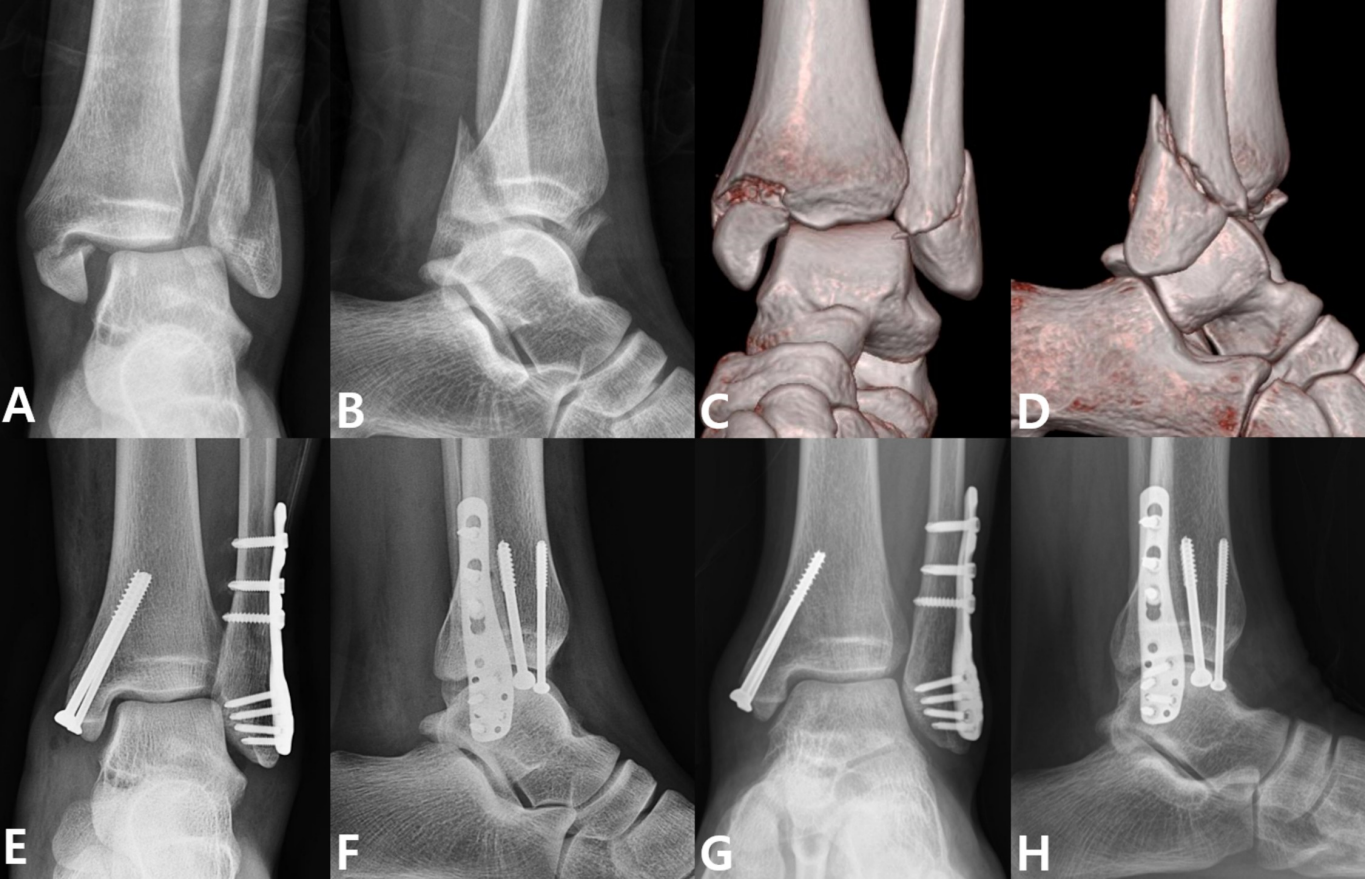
Anteroposterior (A) and lateral (B) radiographs of a supination external rotation ankle fracture. 3D reconstructed anteroposterior (C) and lateral (E) computed tomography images showing an oblique fibular fracture at the syndesmosis level. Anteroposterior (E) and lateral (F) radiographs taken one month after surgery showing anatomical reduction and internal fixation. Anteroposterior (G) and lateral (H) radiographs taken 24 months after surgery showing successful bone union without any perioperative complications.
